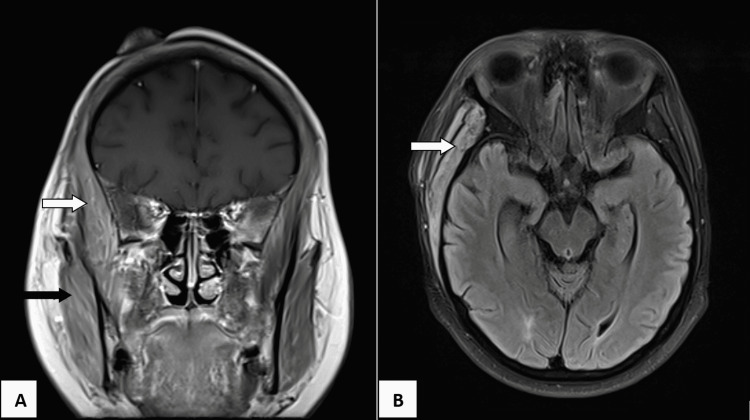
MRI in coronal view (panel A) showing the right temporalis muscle (white arrow) and masseter muscle (black arrow) that are thickened. In axial view (panel B), the right temporalis muscle (white arrow) is thickened with clear plane seen surrounding the muscle. MRI: Magnetic resonance imaging
